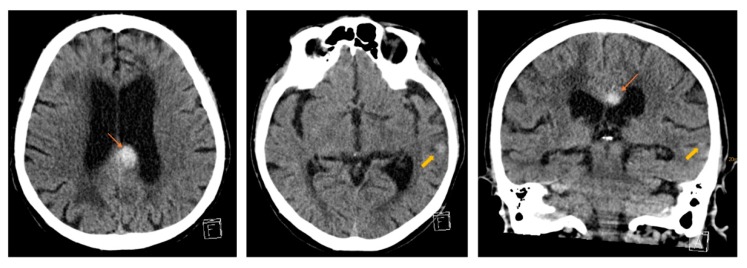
First cranial CT (small arrow—bleeding in the corpus callosum, broad arrow—temporal bleeding).
